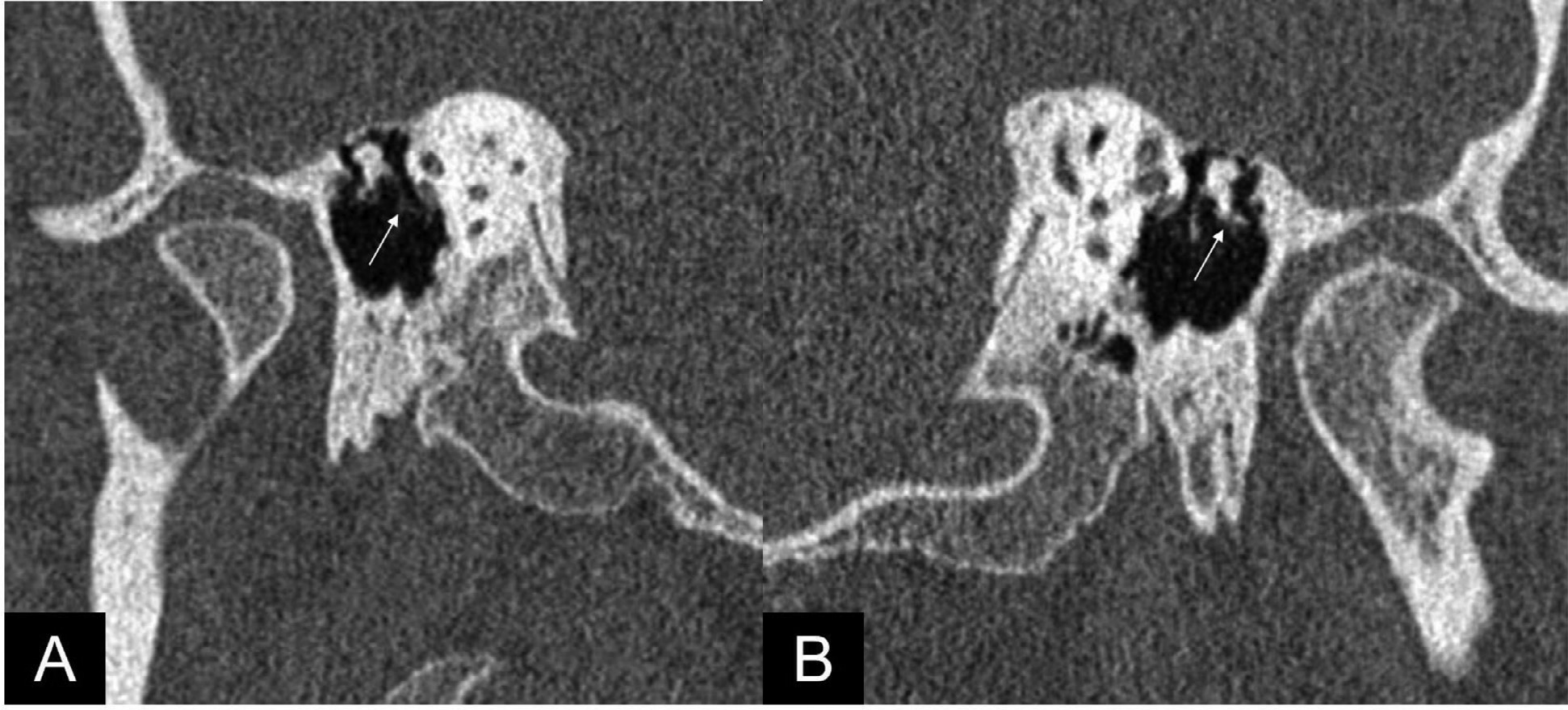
Autoincudotomy in a 34-year-old male presented with conductive hearing loss and otorrhea. High-resolution CT temporal bone with Poschl views (a) right and (b) left reveal loss of mineralization with defective long process of the incus (arrow) on the right side, compared to the left. Minimal hypodense granulation tissue and adhesions were observed.
